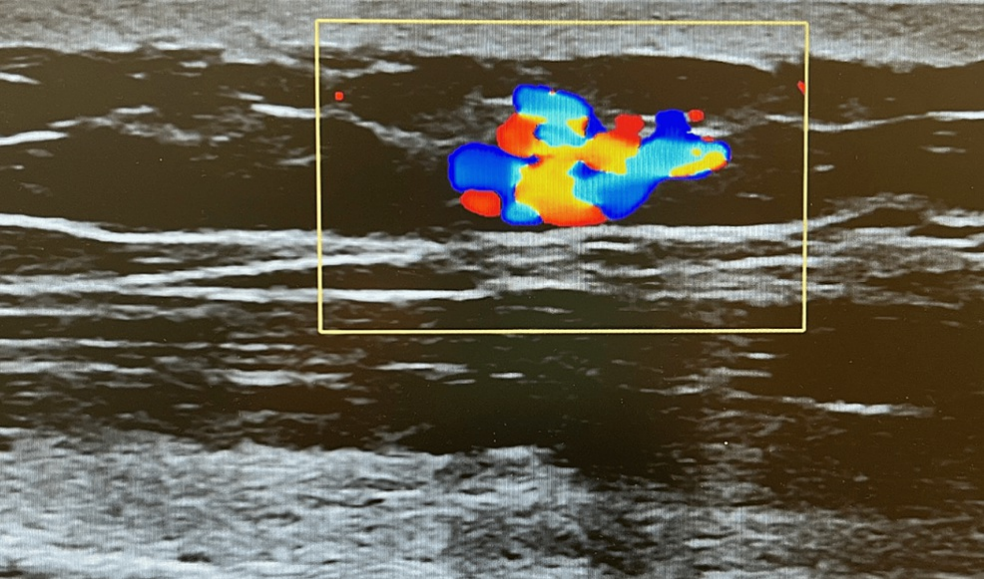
Doppler ultrasonography of the mass shows mixed AV blood flow, leading to a radiologic interpretation of AV fistula due to traumatic sequelae. The yellow box highlights the mixed blood flow seen on Doppler ultrasonography. AV: arteriovenous
